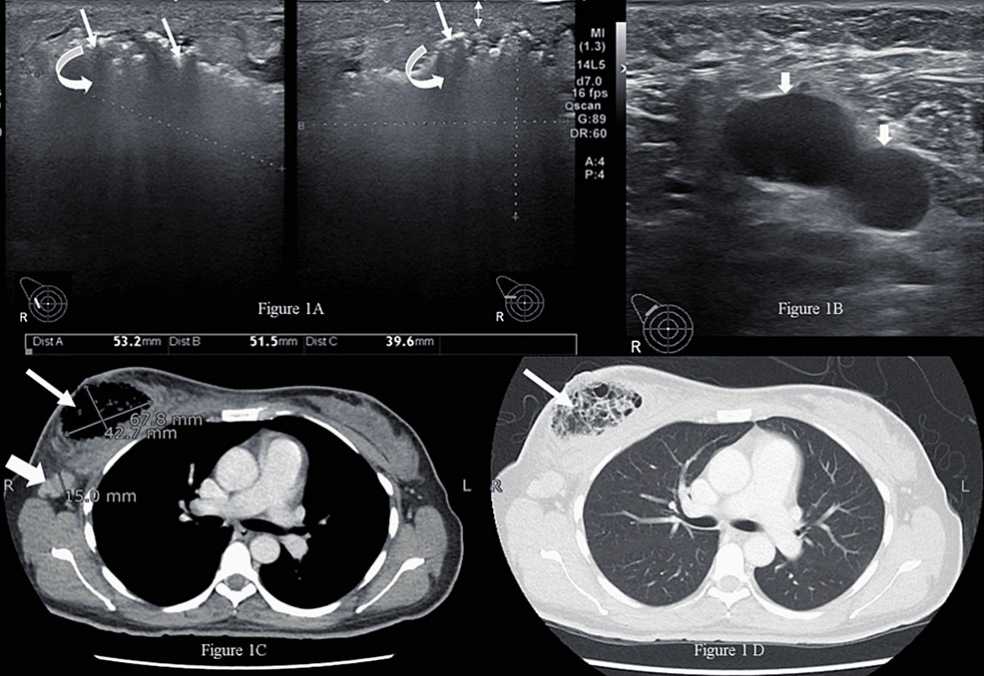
Radiological appearance of the breast lesion (A) Ultrasound image of the right breast upper outer quadrant shows an ill-defined area with echogenic foci representing air (straight arrows) causing dirty shadowing (curved arrows). Skin thickening was also noted (double arrow) (B) Ultrasound of the right axilla shows enlarged hypoechoic lymph nodes (short arrows) with absent hilum (C) Axial contrast-enhanced CT image (mediastinal window) shows the air-containing cavity in the right breast (long thin arrow) and the enlarged right axillary lymph node (short thick arrow) (D) Axial CT image, lung window, at the same level, shows the air-containing right breast cavity with thin internal septations (arrow) CT: computed tomography
